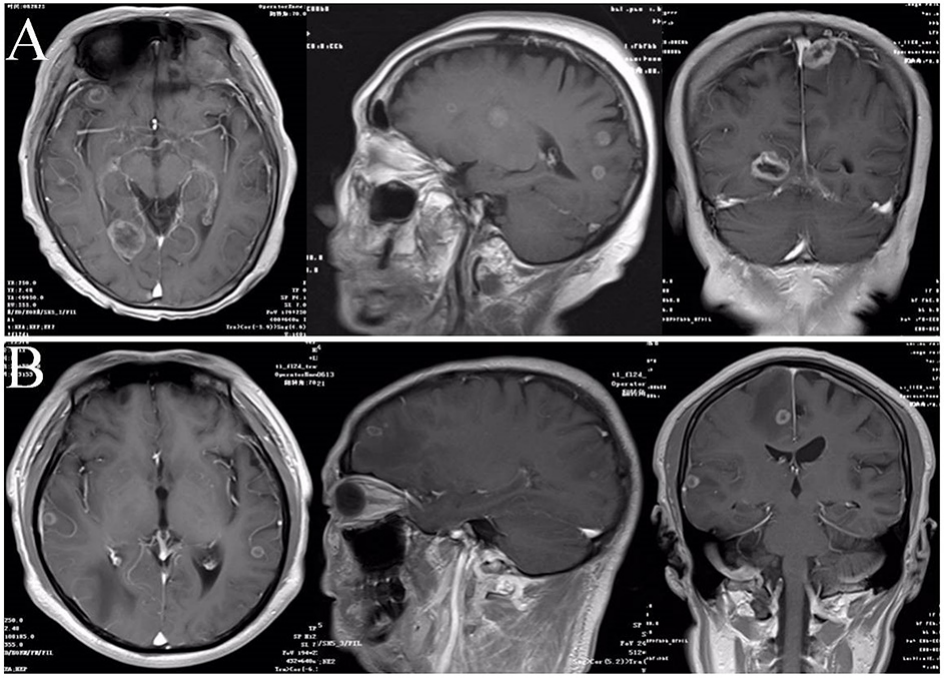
Representative results of brain MRI scans in patients with different EGFR mutation status A. MRI scans in patients with EGFR-mutant. B. MRI scans in patients with wild-type EGFR.
